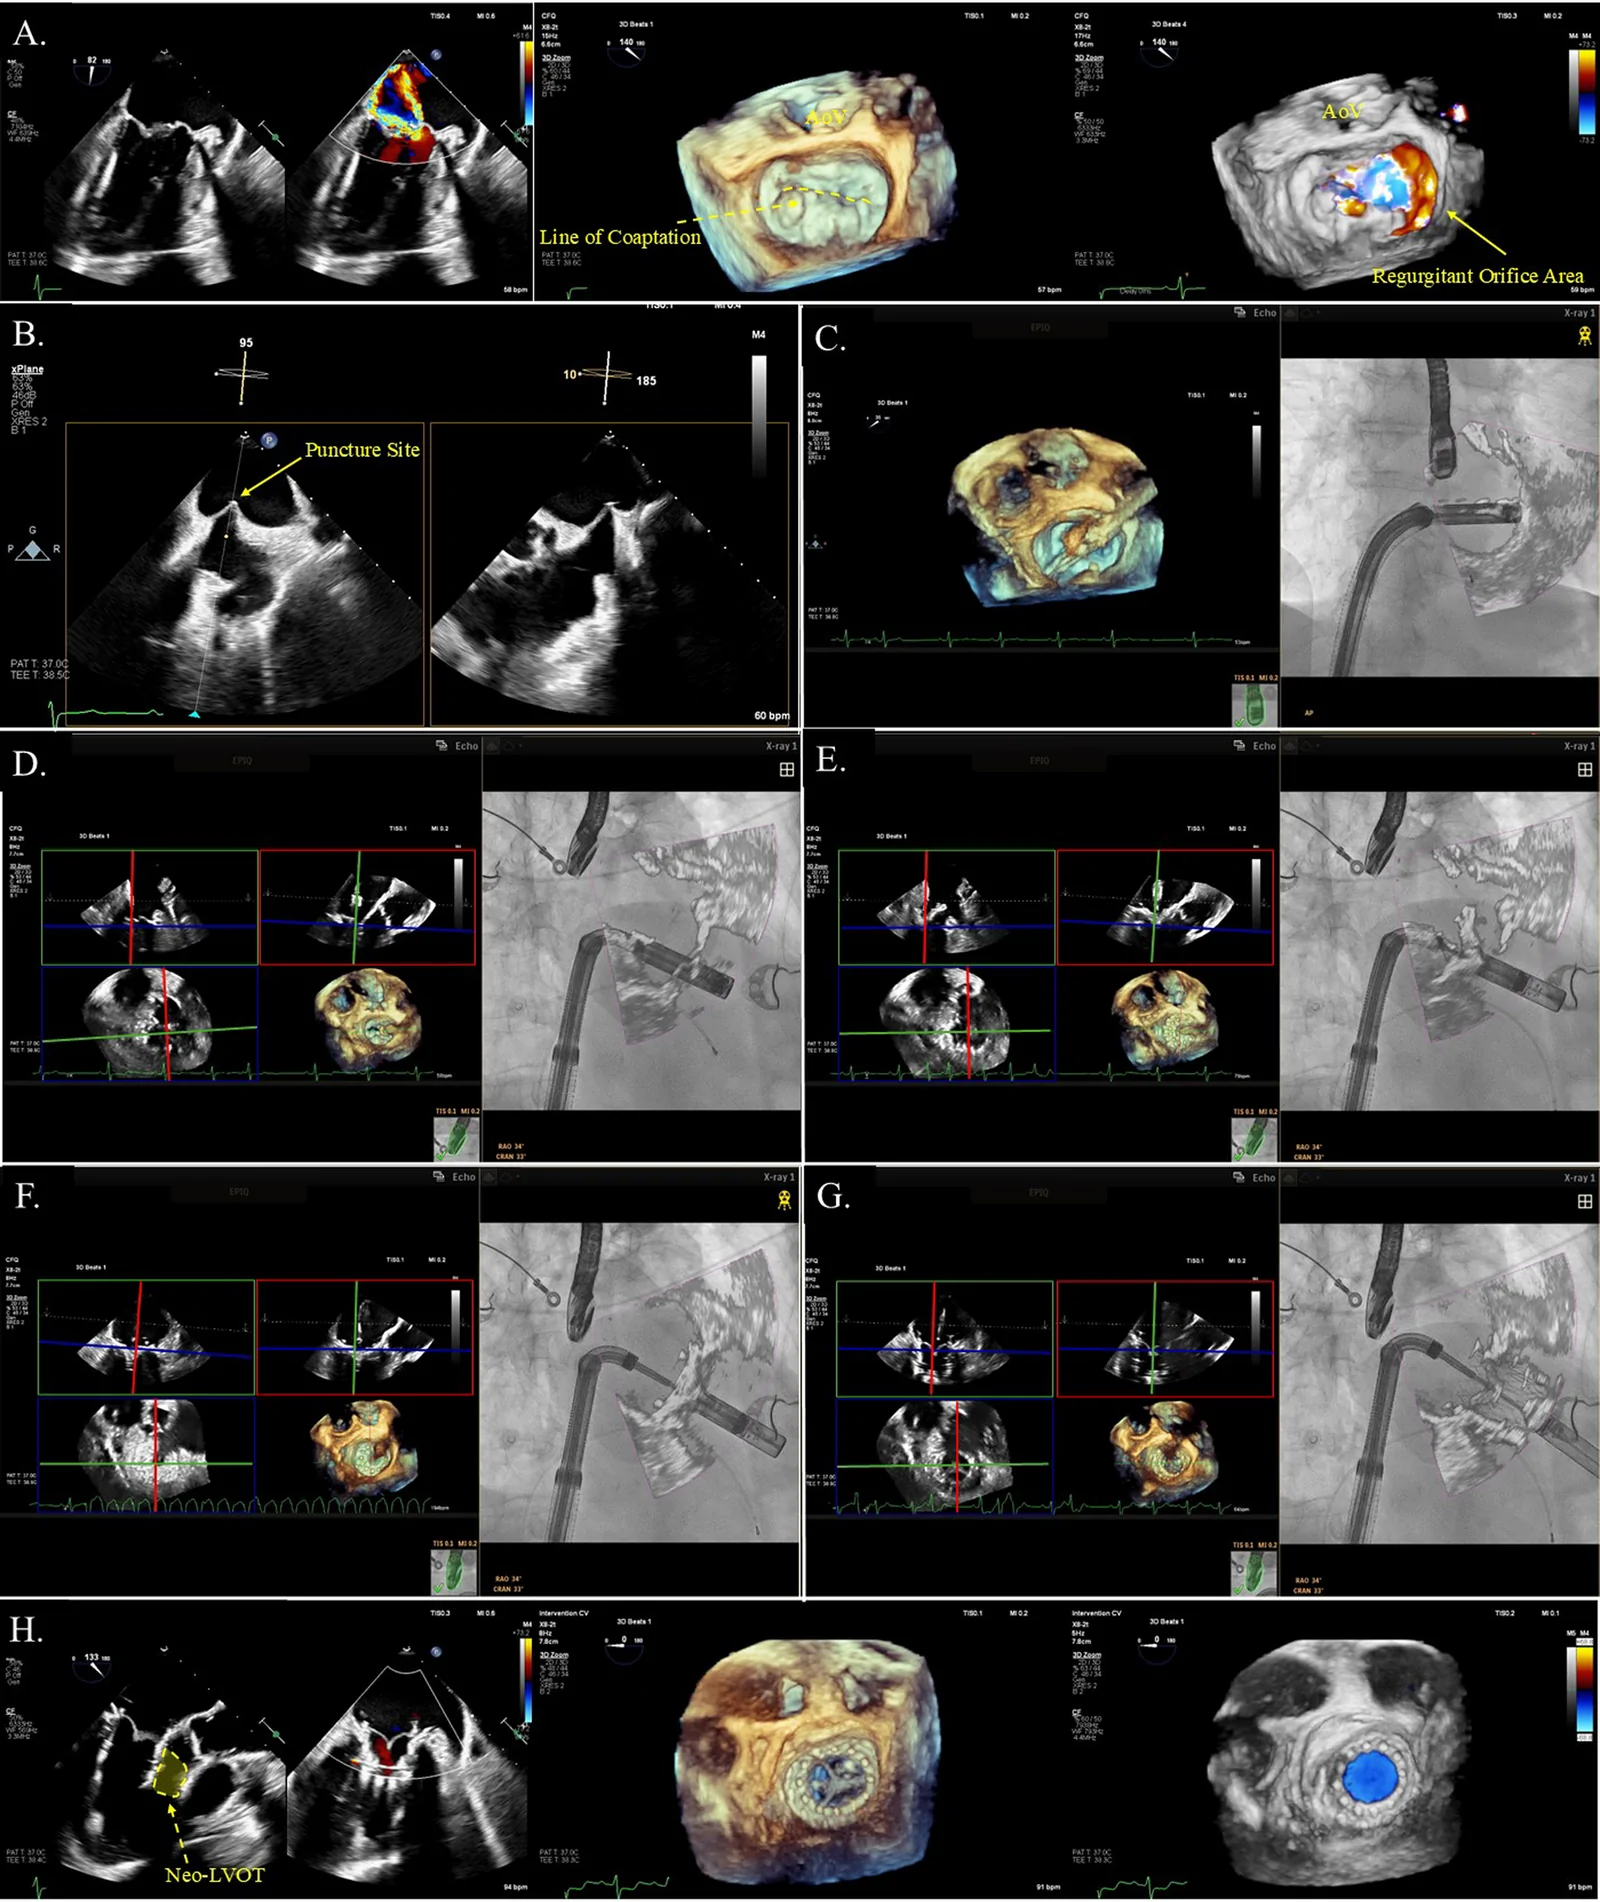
Stepwise multimodal imaging guidance during transcatheter mitral valve replacement (TMVR). A The 2D and 3D transesophageal echocardiography (TEE) demonstrate severe mitral regurgitation (MR) with complex posterior leaflet pathology and significant mitral annular calcification. B The 2D TEE guidance confirming a posterosuperior puncture site with adequate height for large-delivery-system navigation. C The 3D TEE combined with echocardiography-fluoroscopy fusion confirming septal crossing and spatial orientation of the delivery system relative to the annular plane. D Real-time multiplanar reconstruction (MPR) providing orthogonal geometric control during intra-atrial navigation; fusion imaging maintaining a shared spatial reference with fluoroscopy. E, F MPR verification of implantation depth and rotational stability during partial deployment; fusion imaging preserving coaxial orientation. G Integrated MPR and fusion imaging confirming stable annular engagement and appropriate prosthesis seating. H Postdeployment 3D TEE with color Doppler demonstrating complete MR elimination, absence of significant paravalvular leak, and preserved left ventricular outflow tract flow following intrepid TMVR
